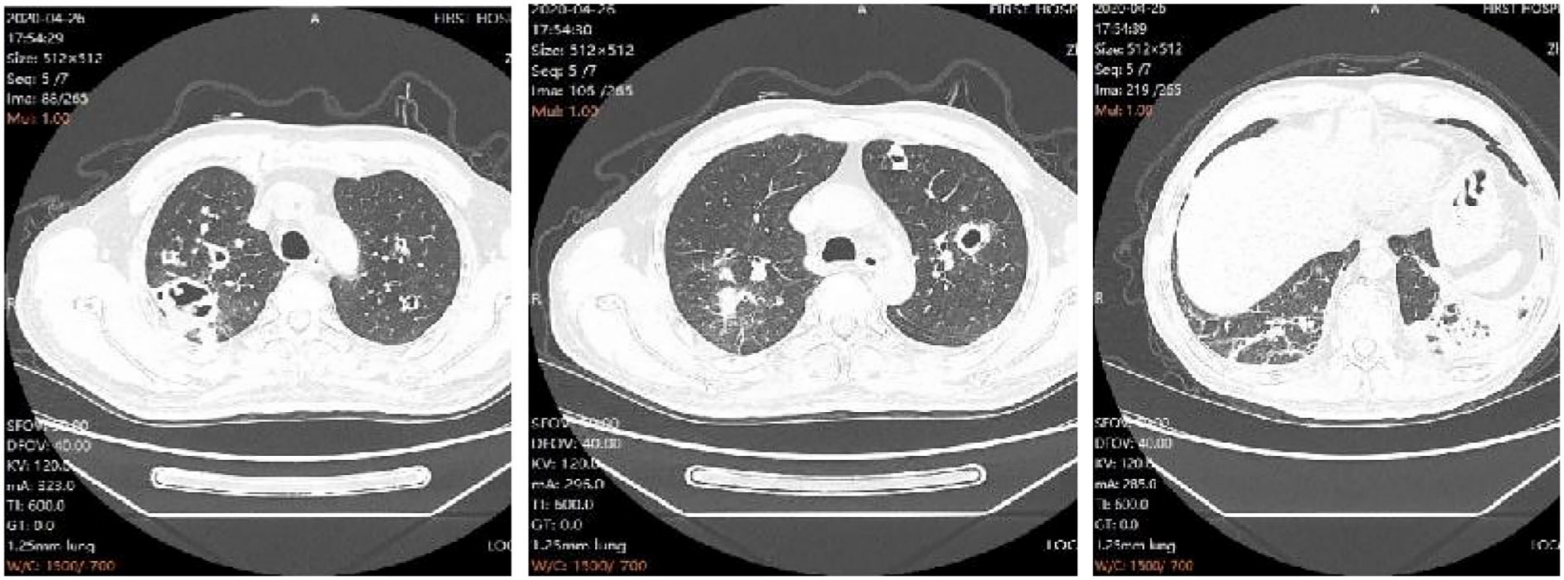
Radiographic progression of lungs.
